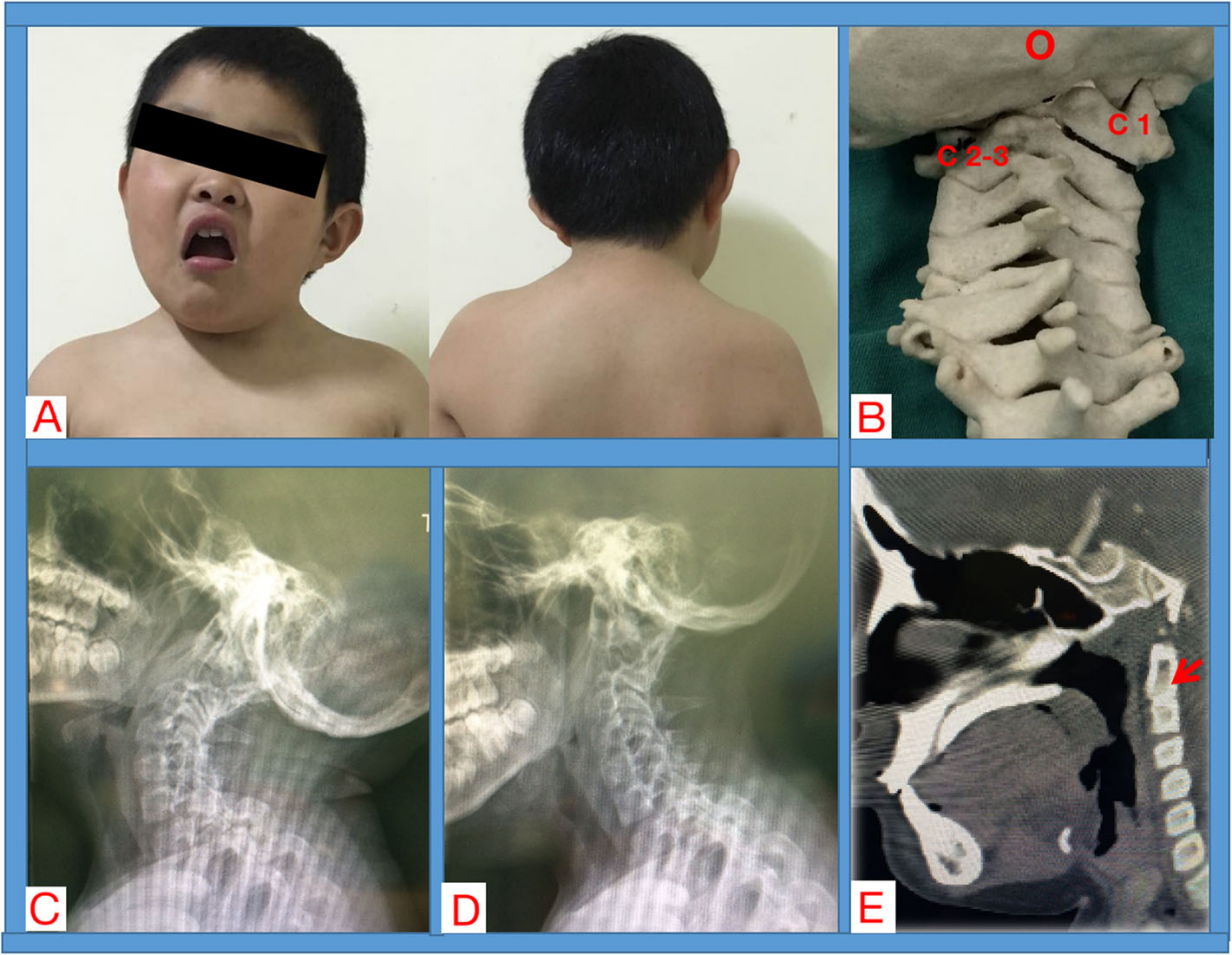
a, preoperative appearance of the pediatric patients with osseous torticollis. b, a Three-dimensional printed cervical vertebrae model of the pediatric patients with osseous torticollis. c, preoperative X-ray imaging of the lateral view of the cervical in extension position. d, the lateral view of the cervical X-ray imaging in reflection position. e, CT imaging showing the cervical spine developmental malformations (red arrow: C2-3 block vertebrae) (C, cervical vertebrae)
